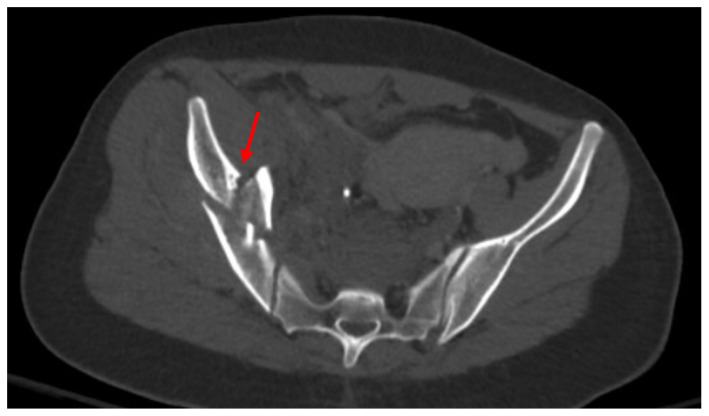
CT abdomen/pelvis images of the described fractures in case 3. Red arrows are used to indicate the location of pelvic fractures for easier identification.
